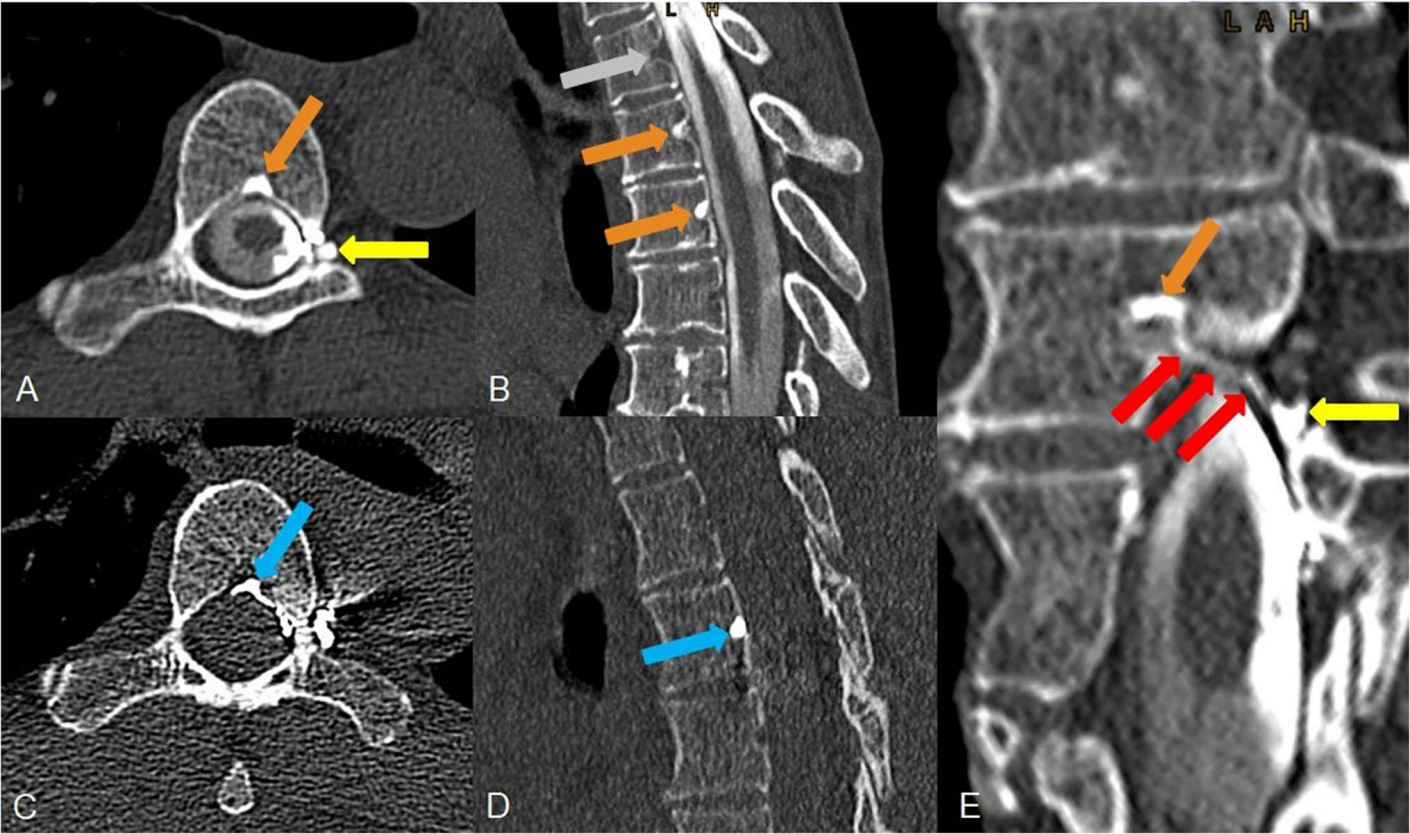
A 55-year-old woman with a central CVF at the level of Th5/6 left. A, B, Left-sided lateral decubitus CTM shows a “hyperdense basivertebaral ein” sign on the level of the CVF and one level above (orange arrows). An associated meningeal diverticulum is located at the left nerve root sleeve (yellow arrow). C, D Post endovascular embolization the Onyx cast can be confirmed in the basivertebral vein in question at the level of Th 5/6 (blue arrow). E, Curved planar reconstruction of the CVF on the CT scan reveals the central drainage from a spinal meningeal diverticulum on the left side (yellow arrow) representing the point of fistula, via the ventral epidural venous plexus at the left side (red arrows) to the proximal basivertebral vein (orange arrow)
